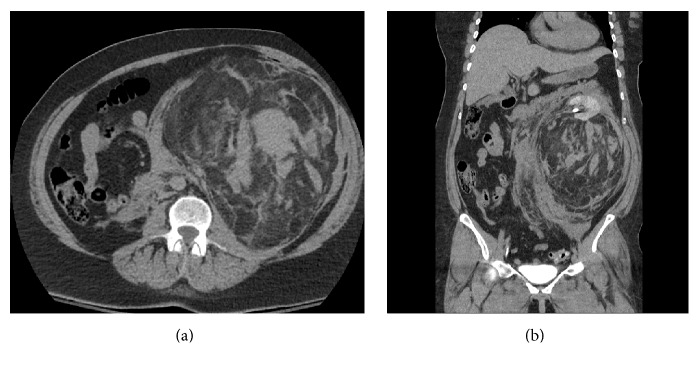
Axial (a) and coronal (b) views of abdominal CT showing massive perinephric AML.
